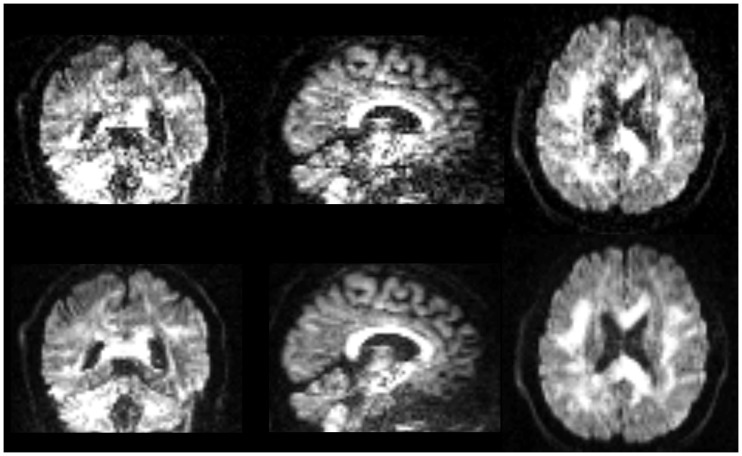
Images are instances of human brain DWI from a given gradient direction, from raw-DWI (first row) and lop-DWI (second row). Raw-DWI was corrected for motion, eddy current, and EPI distortion (see Method).
